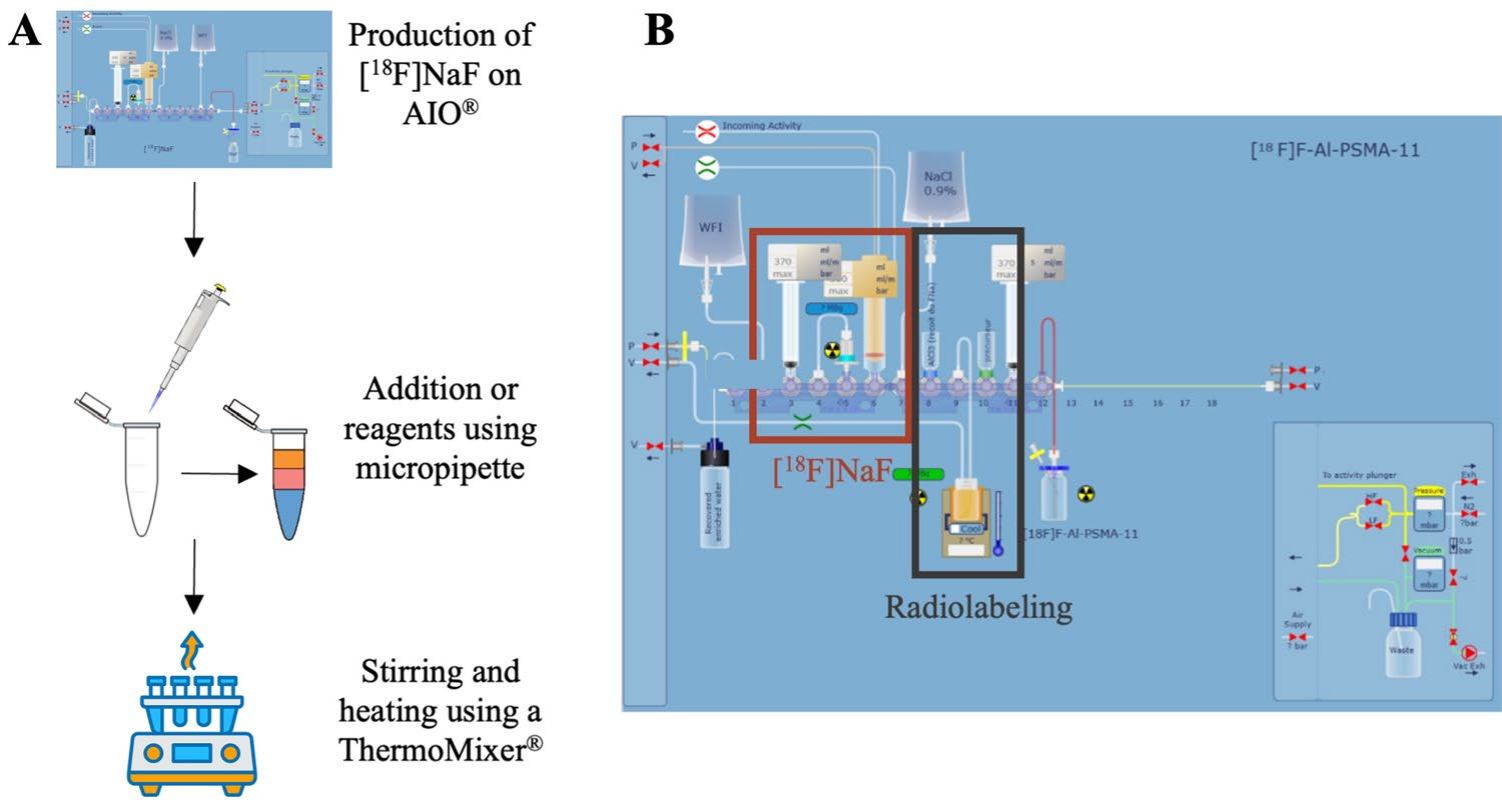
(A) Manual Al$$^{18}$$F-labeling of PSMA-11. (B) Layout of automated Al$$^{18}$$F-labeling of PSMA-11 on AIO^®^ (Trasis).
